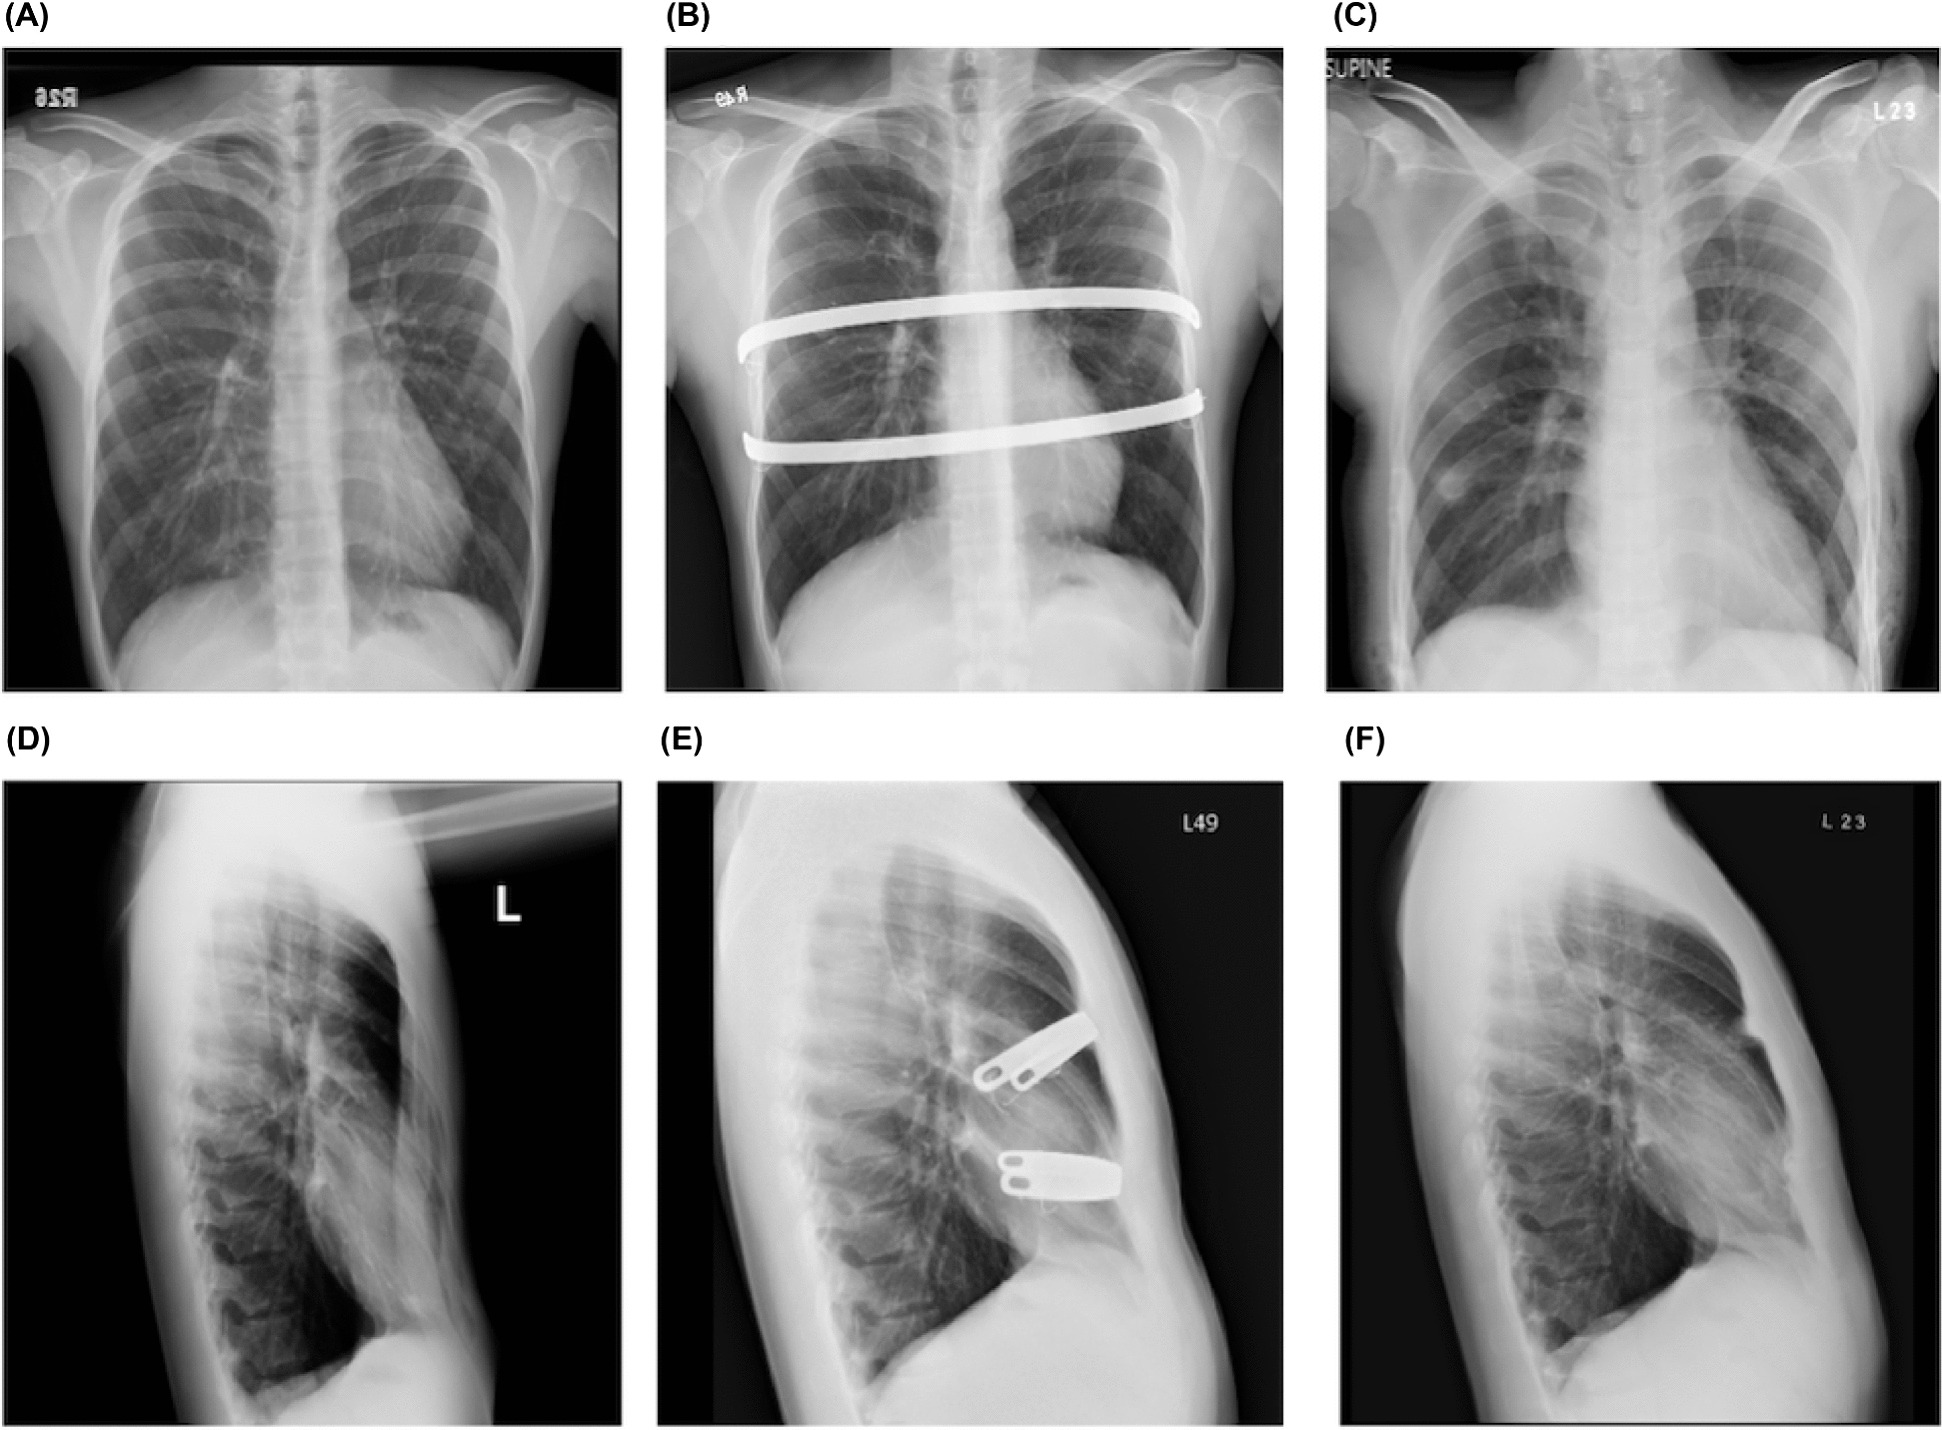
Haller index measurement.  Haller index measurement using posteroanterior and lateral chest radiographs before Nuss repair ( A , D ; CXR-HI: 4.32), before ( B , E ; CXR-HI: 2.35) and after ( C , F : CXR-HI: 2.35) bar removal 4 years post-surgical correction. CXR-HI, Haller index on chest radiography
